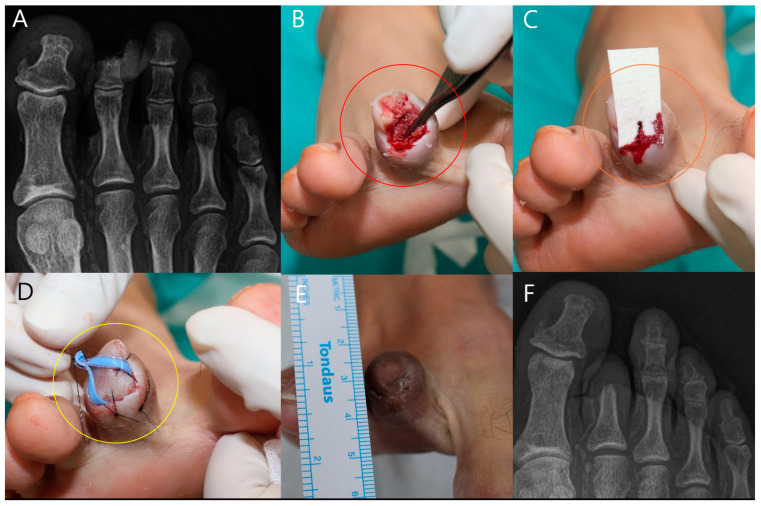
A 62-year-old female patient with a history of uncontrolled diabetes mellitus. (A) Initial X-ray showing bone resorption in the distal phalanx. (B) Intraoperative image after debridement, revealing significant bone exposure (red circle). (C) Single application of micronized acellular dermal matrices (mADMs) applied to the exposed bone (orange circle). (D) Wound closure achieved after mADM application, secured with sutures (yellow circle). (E) Six-week postoperative image showing substantial wound healing and epithelialization. (F) Final X-ray displaying successful bone regeneration and no evidence of osteomyelitis.
